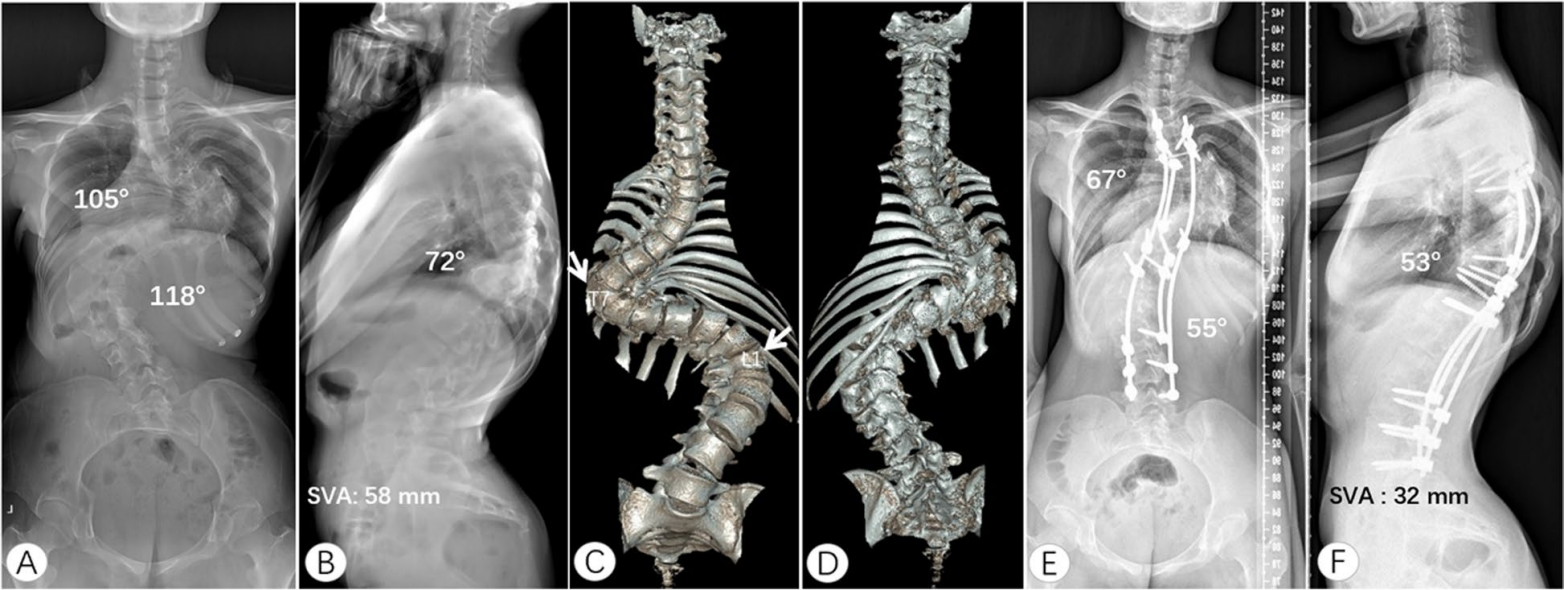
An 18-year-old female patient with rigid congenital kyphoscoliosis. The preoperative thoracic curve, lumbar curve and thoracic kyphosis were 105°, 118° and 74°, respectively. The sagittal plane was imbalance (A, B). Three-dimensional CT showed that the patient had T7 hemivertebra and posterior lamina deformity (C, D). She underwent posterior correction from T3 to L5. Short rods were placed on the apical regions of the thoracic curve and lumbar curve. After a 3-year follow-up, thoracic curve, lumbar curve, and kyphosis were improved to 67°, 55°, and 53°, respectively. The sagittal plane restored balance (E, F). SVA, sagittal vertical axis
